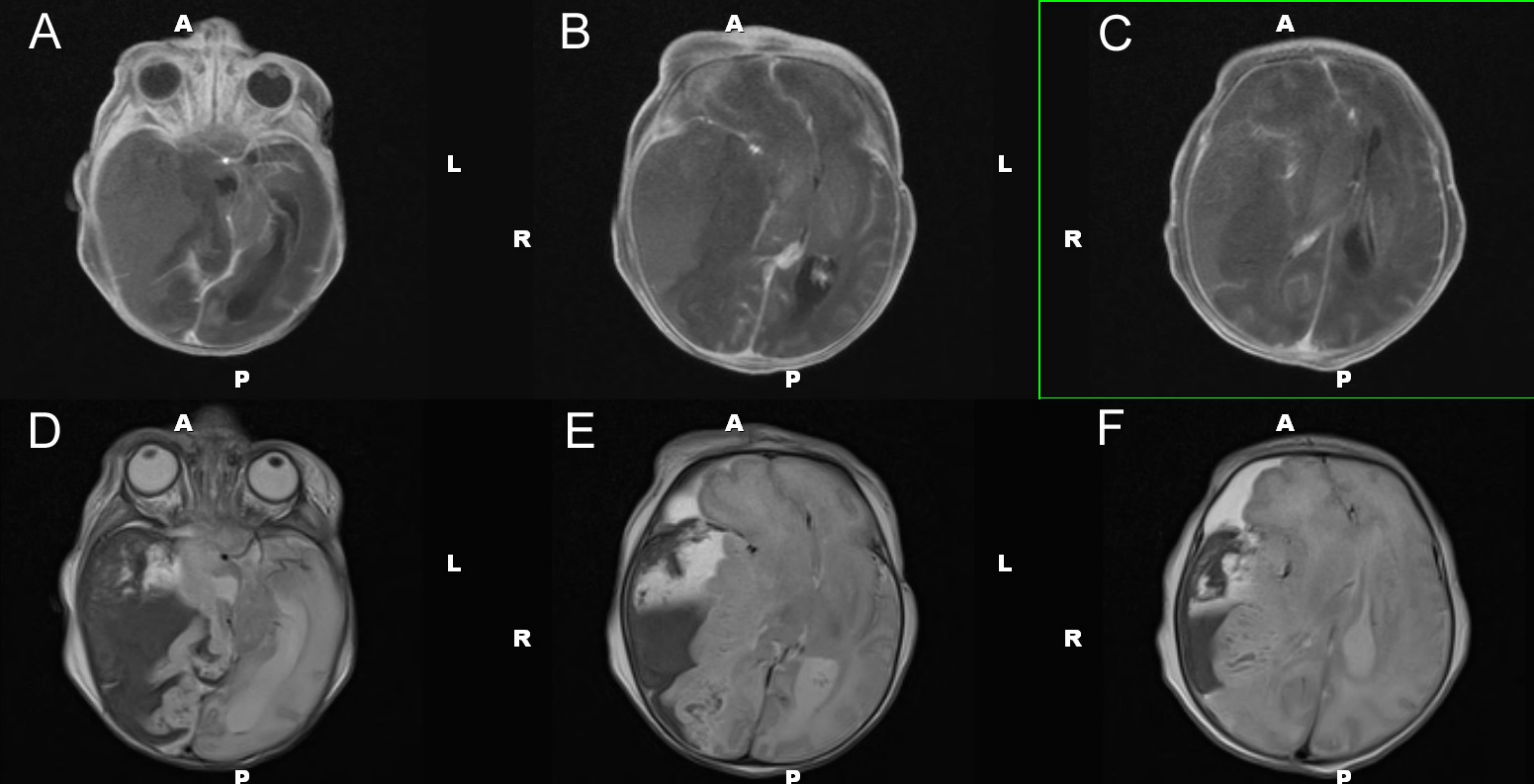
MRI of the brain - T1 with contrast and T2 axial views. T1 with contrast (A-C) and T2 (D-F) axial views characterizing the subdural hematoma.
